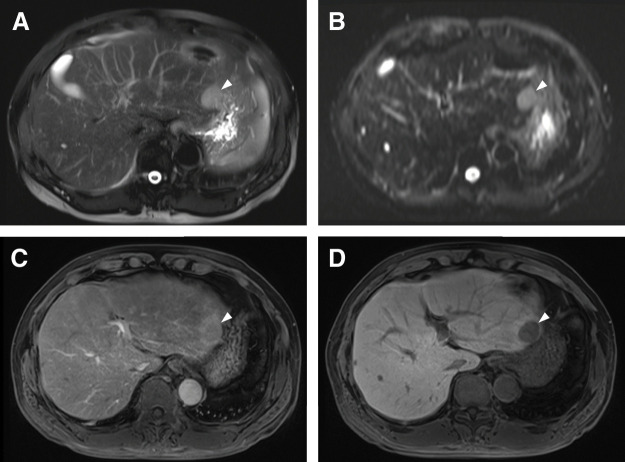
Preoperative MRI findings of the primary hepatic lesion. (A) T2-weighted fat-suppressed HASTE image showed a 3.0-cm hyperintense lesion at the periphery of the left lateral section (arrowhead). (B) Diffusion-weighted image (b = 800 s/mm^2^) showed the lesion as hyperintense (arrowhead). (C) Arterial-phase dynamic contrast-enhanced MRI showed mild hyperenhancement of the lesion (arrowhead). (D) In the hepatobiliary phase obtained 20 min after contrast administration, the lesion appeared hypointense relative to the surrounding liver parenchyma (arrowhead). HASTE, half-Fourier acquisition single-shot turbo spin echo
